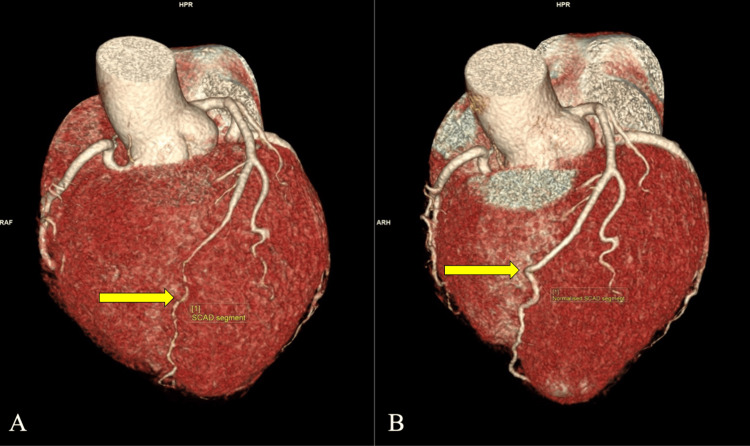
A: Reconstructed three-dimensional VRT image from coronary CT angiography showing type 2A SCAD affecting the LAD artery upon admission (arrow); B: Follow-up imaging after eight months, revealing resolution of the affected segment (arrow). VRT: Volume rendering technique; SCAD: Spontaneous coronary artery dissection; LAD: Left anterior descending.
